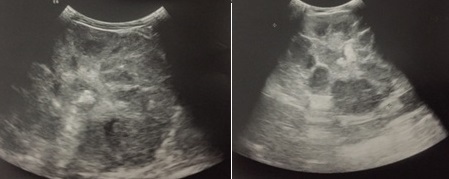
Echographie du rein: vue coronale avec calcification sur un parenchyme hypoéchogène par rapport au rein droit avec multiples zones hypoéchogènes sans vascularisation Doppler et dilatation pyélique avec parois épaissies et nombreux échos intraluminaux
